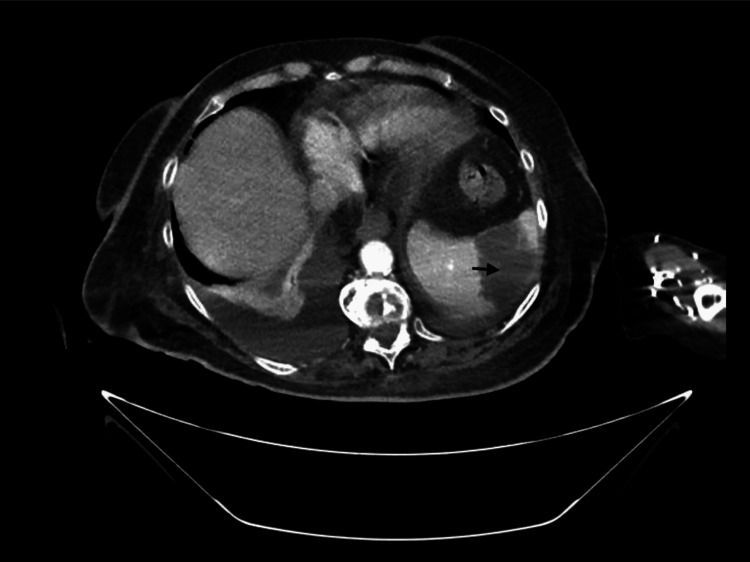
Contrast-enhanced abdominal CT scan demonstrates a triangular area of hypodensity (arrow) in the spleen consistent with splenic infarction CT: computed tomography
